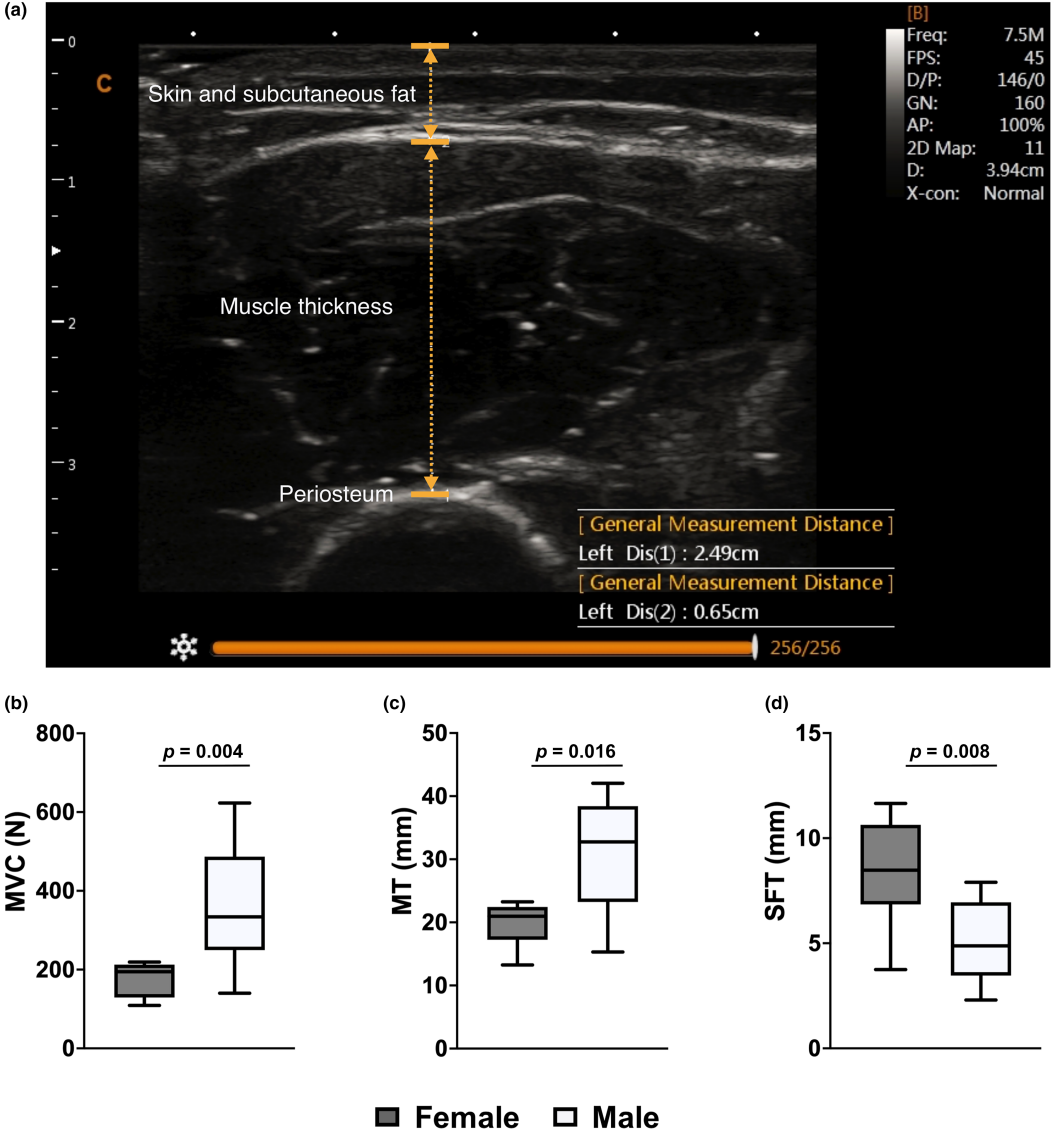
(a) Ultrasonography real‐time measurement of muscle thickness and SFT of biceps brachii from one ultrasound scan for one male participant. In this example, MT was measured to be 24.9 mm while SFT was measured to be 6.5 mm. Group data (*n* = 10 for males and *n* = 8 for females) for (b) MVC force, (c) bicep brachii MT, and (d) overlying biceps brachii SFT. Data presented as box and whisker plots. MVC, maximum voluntary contraction; MT, muscle thickness; SFT, subcutaneous fat thickness.
